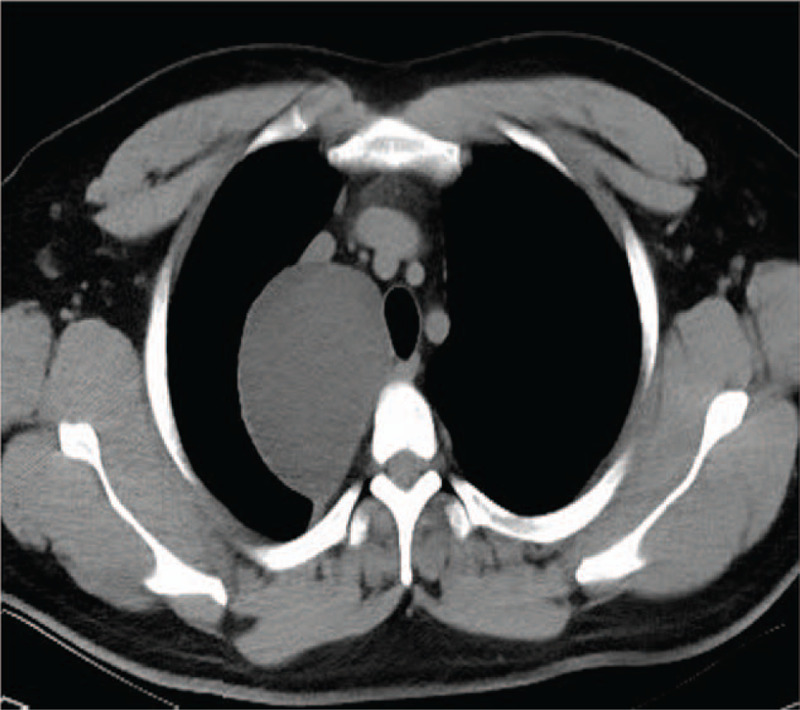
Chest computed tomography (CT) showed a thin wall cystic lesion with the size of 71 mm × 81 mm located in the posterior superior vena cava of the right superior mediastinum.
